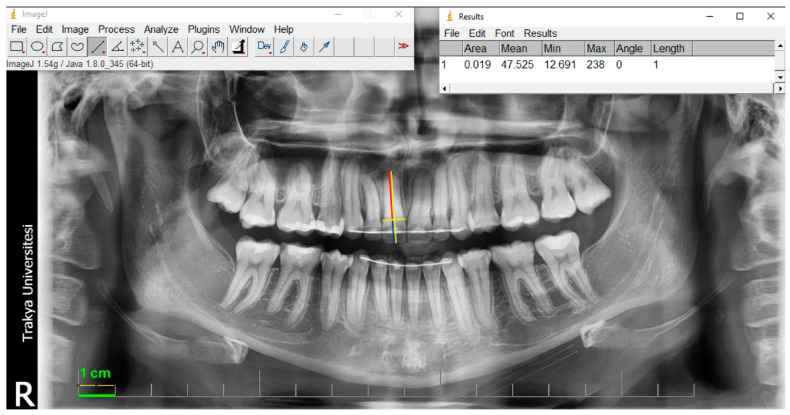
Measurements are taken from a panoramic radiograph. The cementoenamel junction and the long axis of the tooth are represented by yellow lines. The blue line shows the length of the crown, and the red line shows the length of the root.
